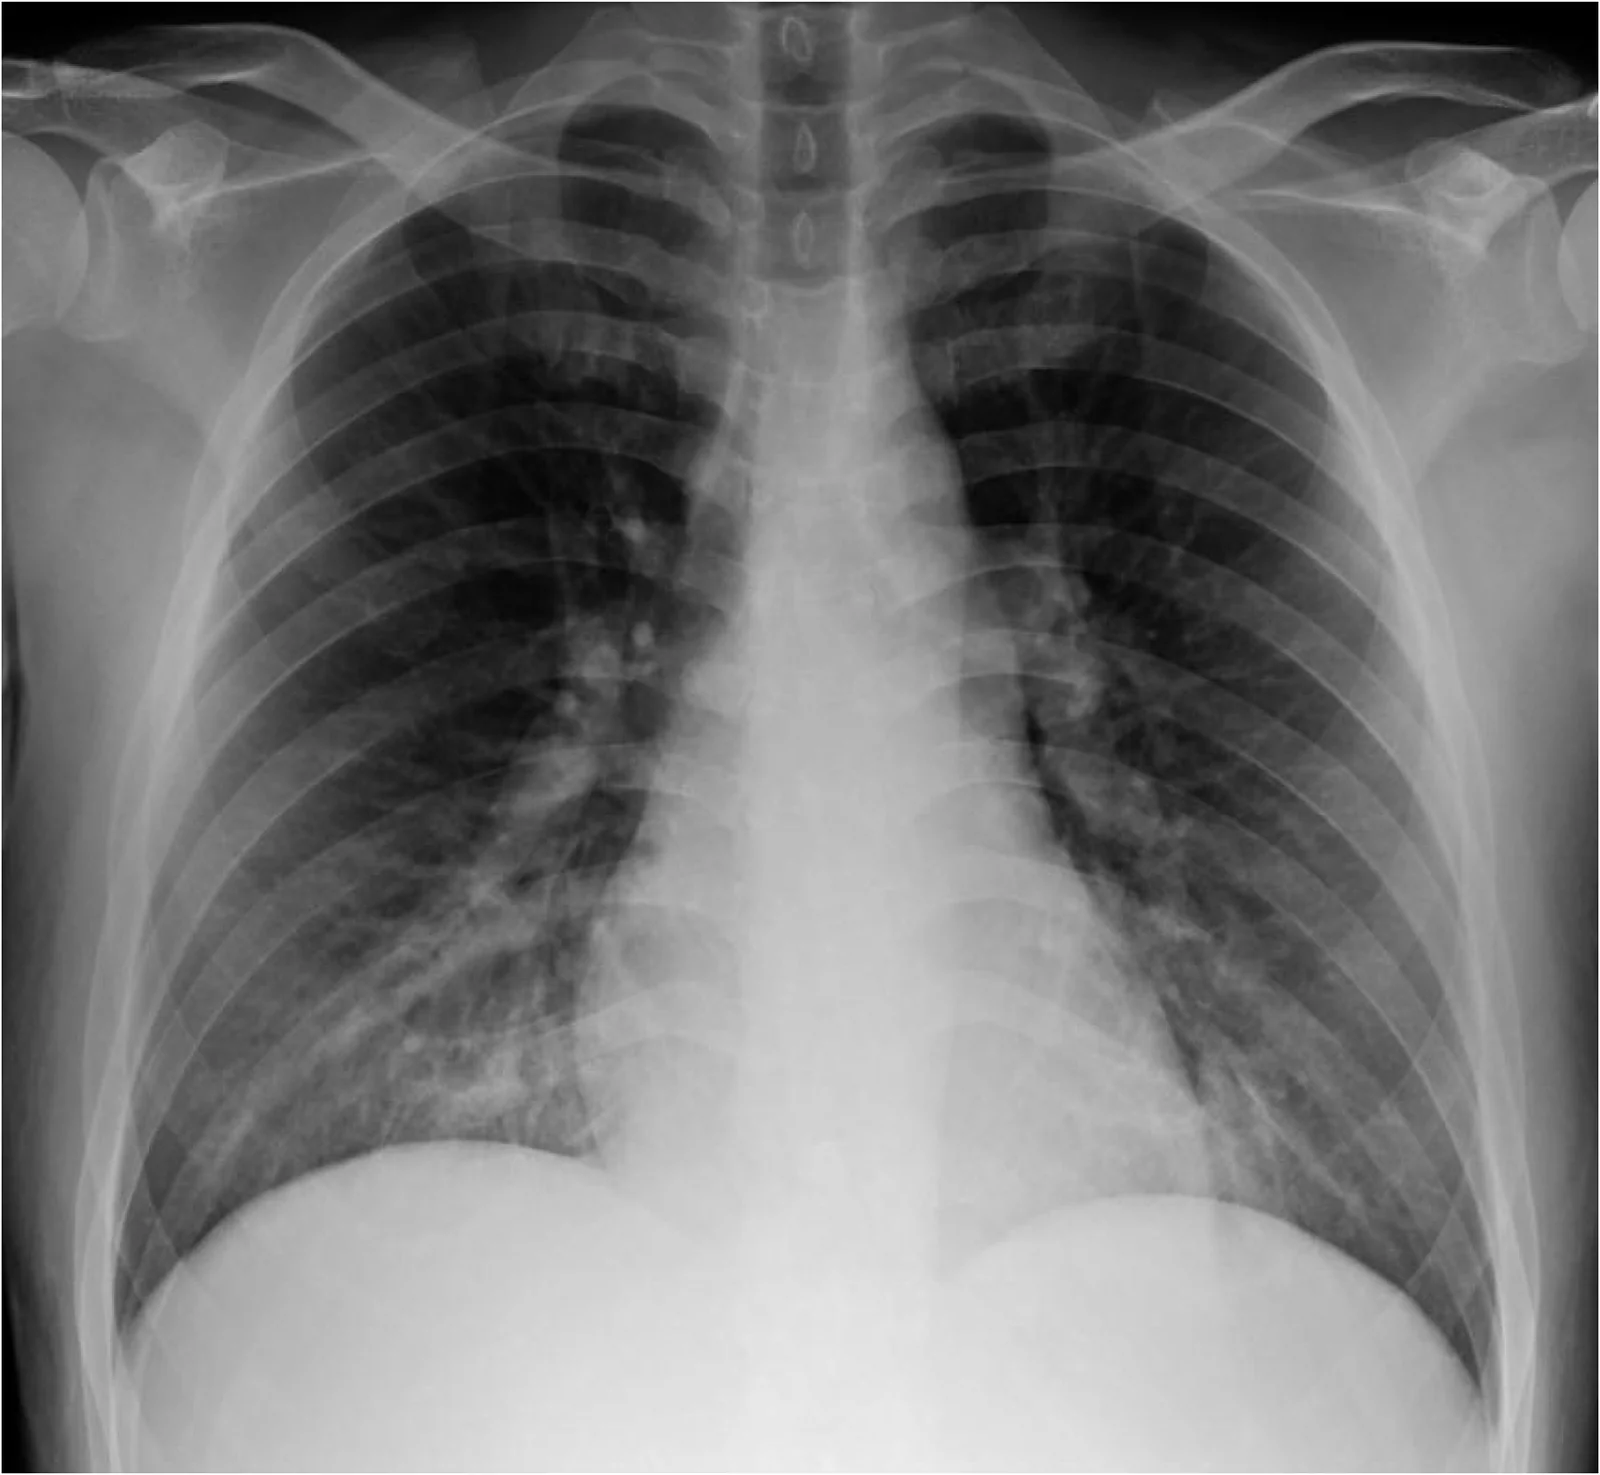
Chest radiograph demonstrating bilateral basal infiltrates and opacities corresponding to the septic pulmonary emboli demonstrated on chest CT (Fig. 2A).
